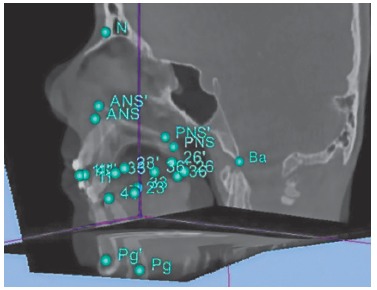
Display of the three planes (sagittal, axial and coronal) of the registered pre- and postoperative CBCTs, with 3D distribution of landmarks on 3D Slicer 3,6 software.
